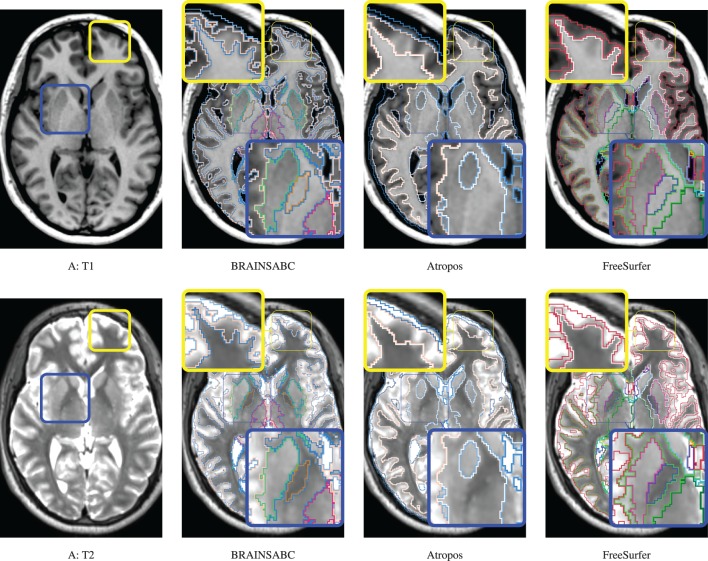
Visual Comparison of Scan A in Table 2. Tissue classification results from three applications, BRAINSABC, Atropos of ANTS Tools, and FreeSurfer on top of T1- and T2-weighted images, multi-modal repeated scan processing. This subject present relatively normal tissue ratio, reflecting minimal, if exists, atrophy in brain tissues. Red and blue boxes highlight where tissue classification is more differentiated from each other. BRAINSABC were highly agreeable to Atropos and FreeSurfer in cortical area in general. For subcortical area (blue box), however, BRAINSABC produced more agreeable to FreeSurfer than Atropos. Atropos were the most conservative in the subcortical GM identification among three methods. For CSF (or ventricle) region, which is more distinguishable from T2-weighted modality, BRAINSABC produced very robust results across scans.
